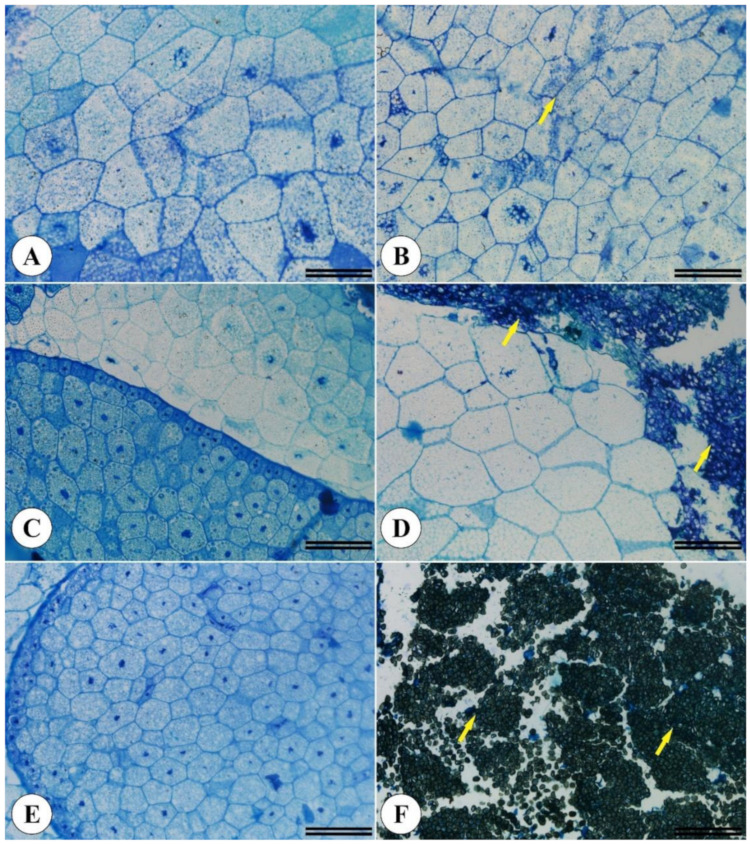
Morphoanatomical *Brachiaria brizantha* seed structures. Seeds previously selected by the X-ray test displaying no filling alterations (A,C,E) and filling alterations (B,D,F). (A,B) Marandu cv. (C,D) Piatã vc. (E,F) Xaraés cv. A total of 200 seeds from each cultivar were analyzed. Yellow arrows indicate cellular changes.
